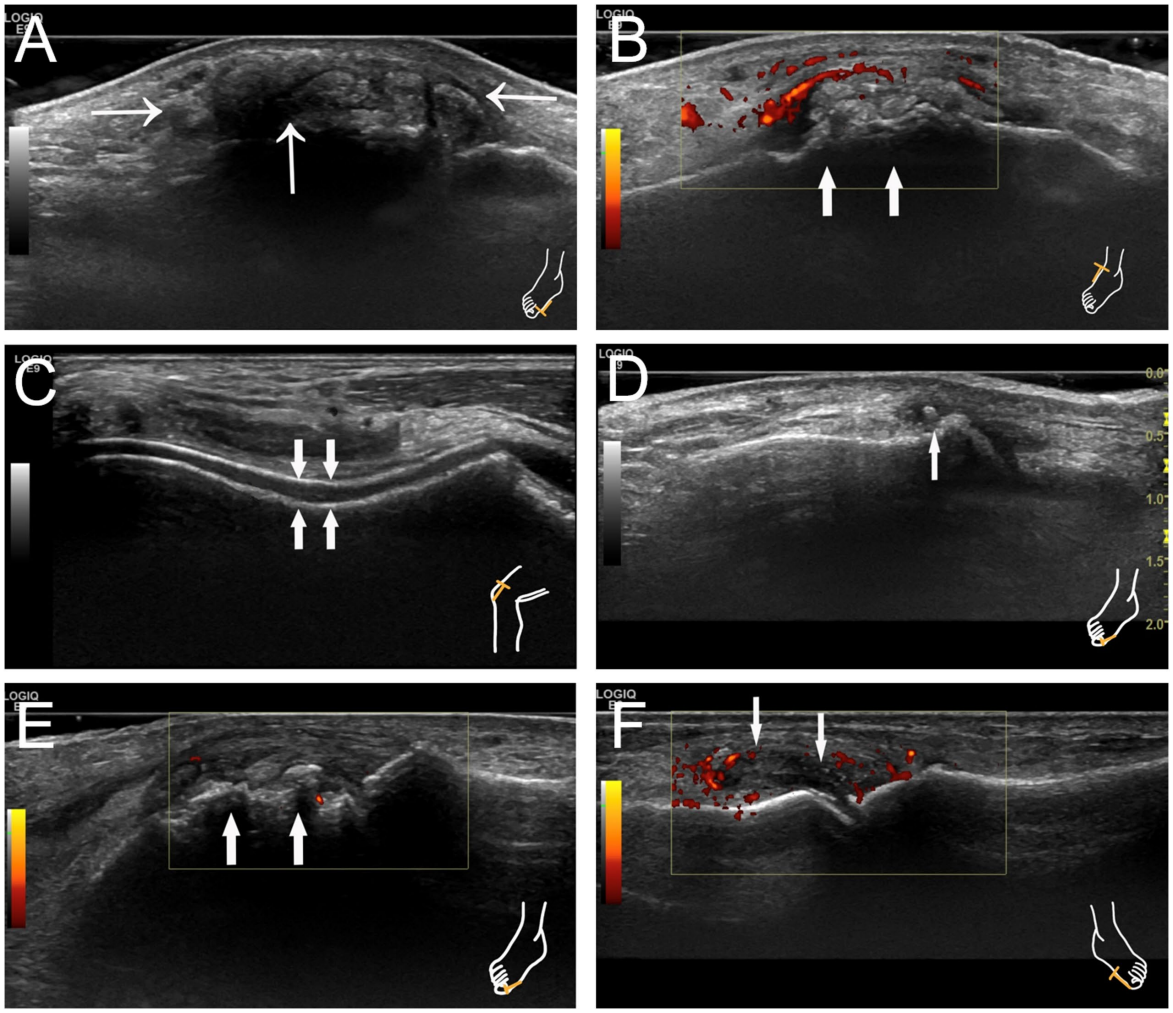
Characteristic images of joint ultrasonography in patients with gout. (A) Tophus with posterior acoustic shadow at the right first metatarsophalangeal joint indicated by thin arrows. (B) Tophus with hyperechoic echo at the right knee indicated by thick arrows. (C) The double-track sign at the right knee indicated by thick arrows. (D) Hyperechoic aggregates at the right first metatarsophalangeal joint indicated by a thin arrow. (E) Bone erosion at the right first metatarsophalangeal joint indicated by thick arrows. (F) Synovial hyperplasia at the left first metatarsophalangeal joint with synovitis indicated by thin arrows.
